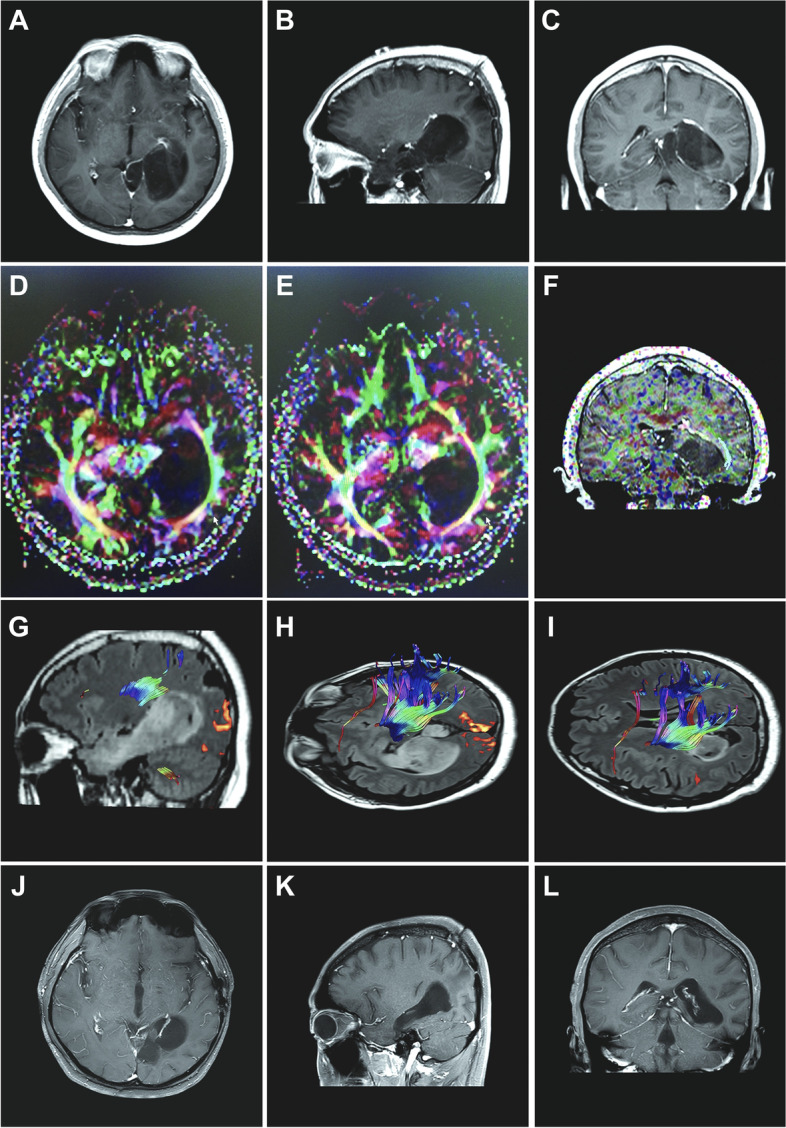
Left temporal-occipital-parietal giant glioma resection via temporoparietal combined keyhole approach. A–C Pre-operation MR images, D–F pre-operation DTI demonstrating the relative location between optic radiation and tumor, G–I pre-operation relative location between visual center, pyramidal tract, and tumor, and J–L postoperation MR images
